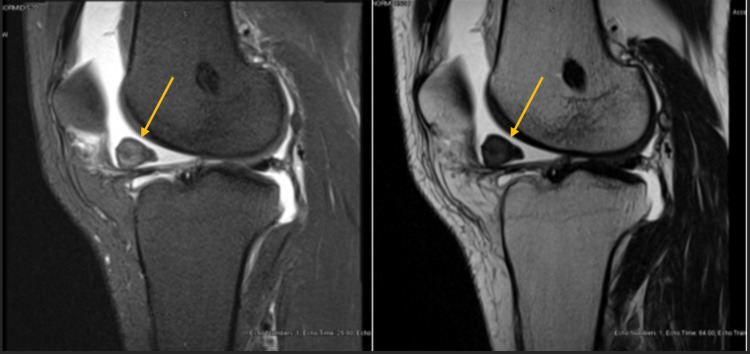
T2 (left) and T1 (right) MRI sagittal images of the left knee showing a heterogeneous foreign lesion over the anterior portion of the knee
